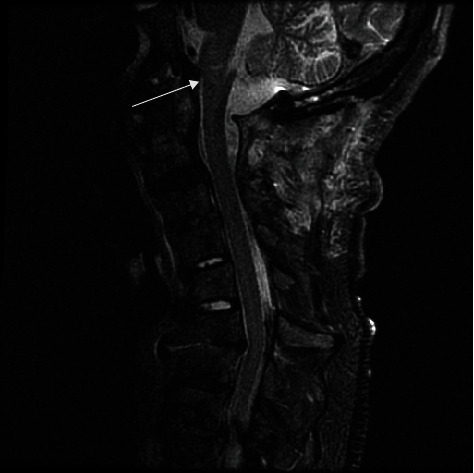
Postoperative MR cervical T2-weighted sagittal image demonstrates resolution of the cervicomedullary compression and caudal medullary cyst.
